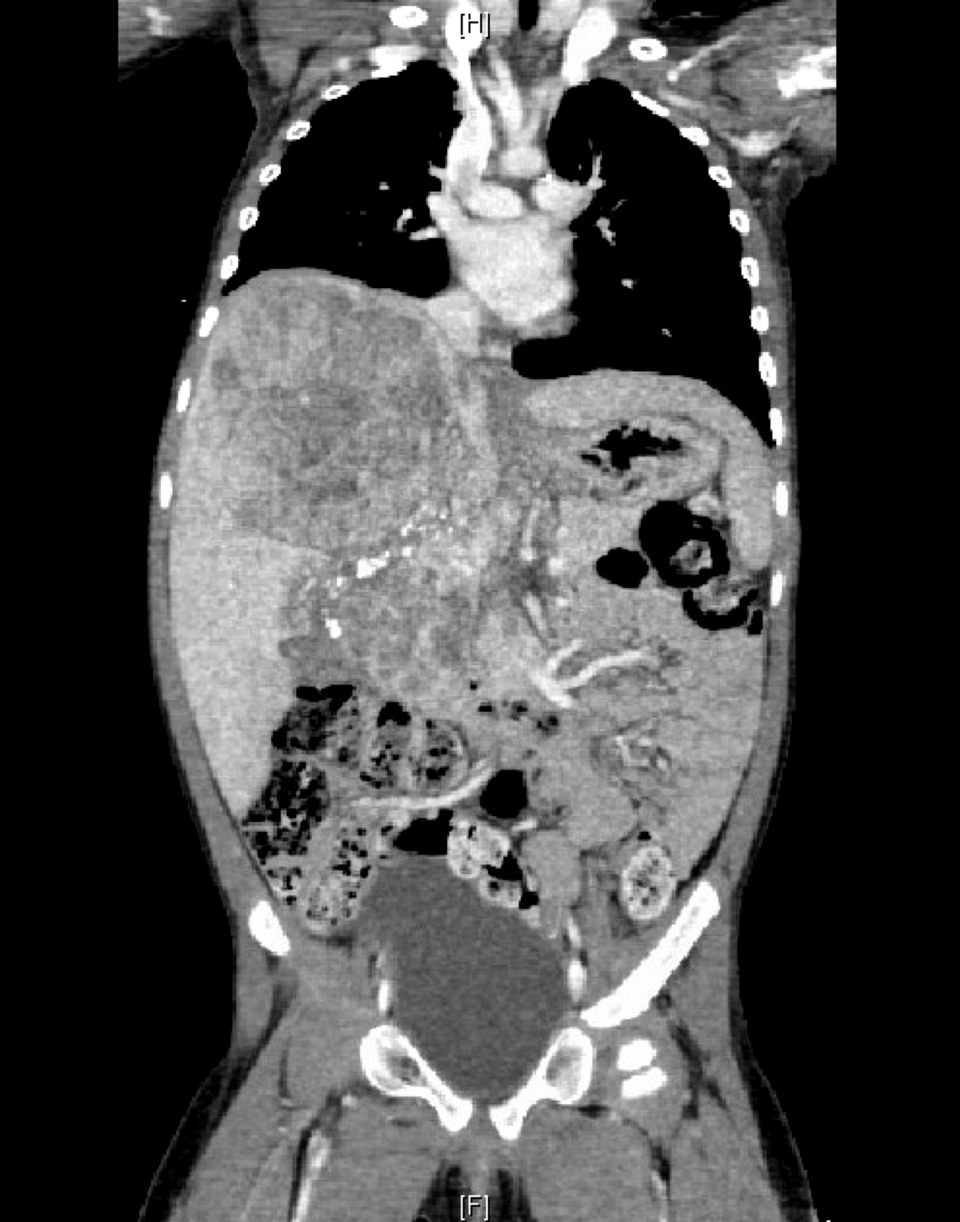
First CT scan two weeks following TARE Y90 treatment demonstrating decreased mass effect of the hepatoblastoma. CT: computed tomography; Y90: yttrium-90; TARE: transarterial radioembolization
